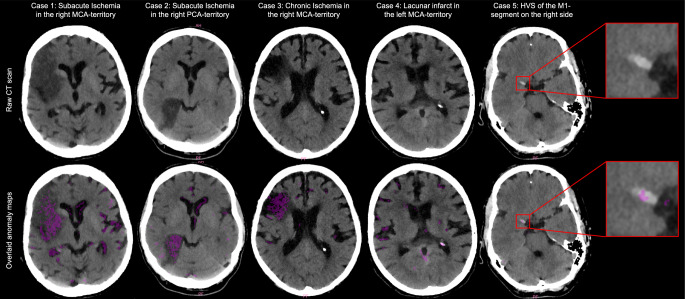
Case examples for correct detection and patient labeling in subacute ischemia (cases 1 and 2), chronic ischemia (Case 3), lacunar DWM infarcts (Case 4) and HVS (Case 5). Shown are the raw CT scans (*top panel*) with the corresponding anomaly maps (*lower panel*, *segmentations of anomalous findings in pink*). *DWM* deep white matter, *HVS* hyperdense vessel sign, *MCA* middle cerebral artery, *PCA* posterior cerebral artery
